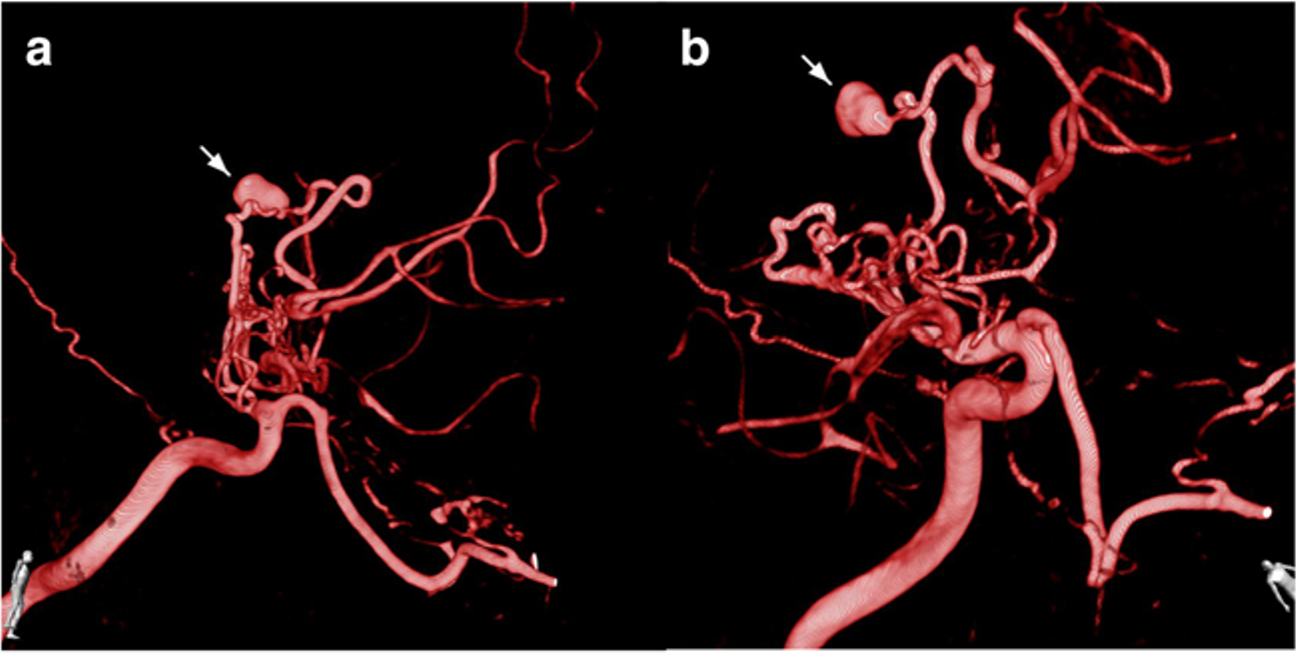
The 3D reconstruction of the follow-up CT angiogram showing the configuration of the collateral vessel aneurysm
